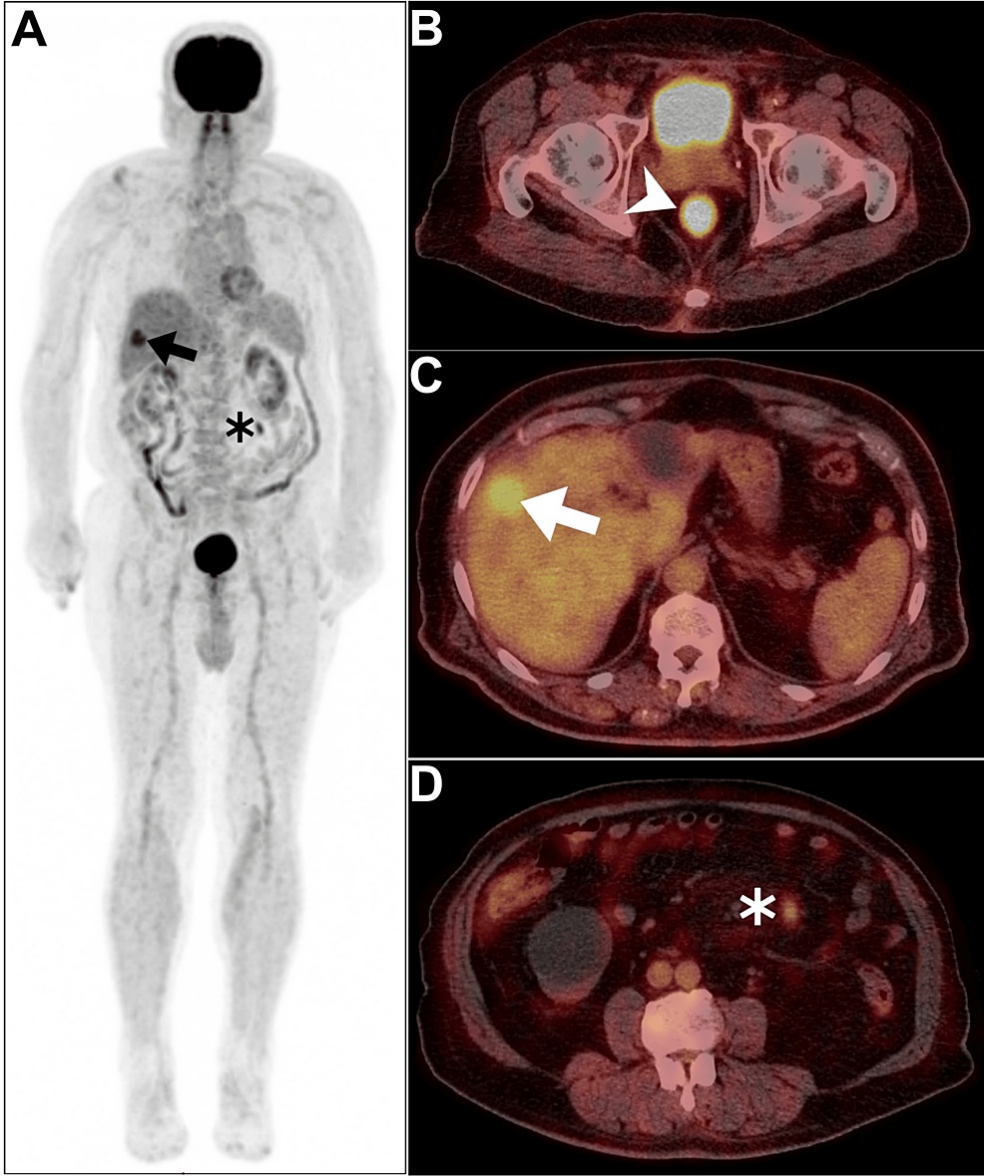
Follow-up PET/CT imaging performed following the sixth cycle of cetuximab maintenance A: Maximum-intensity projection image revealing evidence of two hypermetabolic foci, one of which occupies the liver (arrow), and the other one appears within the peritoneal cavity (asterisk). B: Axial rectal PET/CT image demonstrating more prominent hypermetabolic primary rectal disease (arrowhead). C: Axial hepatic PET/CT image revealing more prominent hypermetabolic hepatic hypodensity indicative of a progressive metastatic liver lesion (arrow). D: Axial abdominal PET/CT image showing interval development of a newly seen hypermetabolic peritoneal deposit (asterisk).
